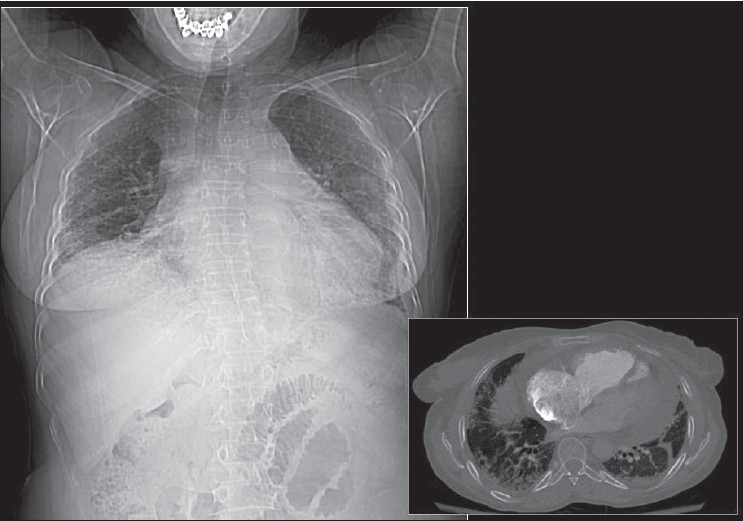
Pericardial effusions may not be that obvious on a chest radiograph. A CT scout film (left) shows nonspecific cardiomegaly in a patient with a clinical diagnosis of viral myocarditis. An axial CT section through the mediastinum shows a moderate-sized pericardial effusion
